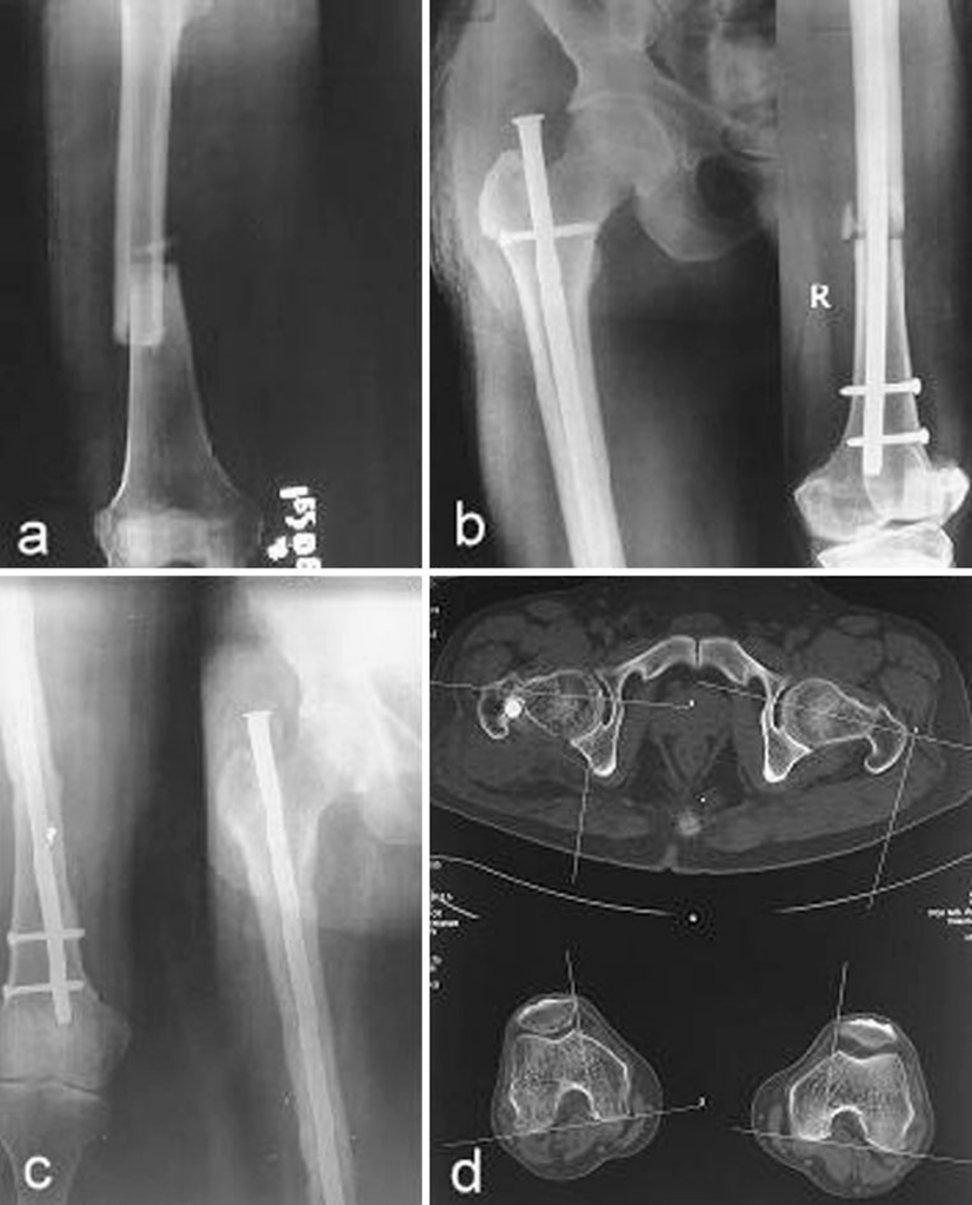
a Preoperative anteroposterior radiograph of thigh showing AO type B2 fracture and type I fracture according to Winquist–Hansen classification. b Immediate postoperative anteroposterior radiograph showing stabilization of fracture with interlocking nail. c Dynamization by removing proximal screw was performed to achieve union. d CT scan showed external rotational deformity of 21°
